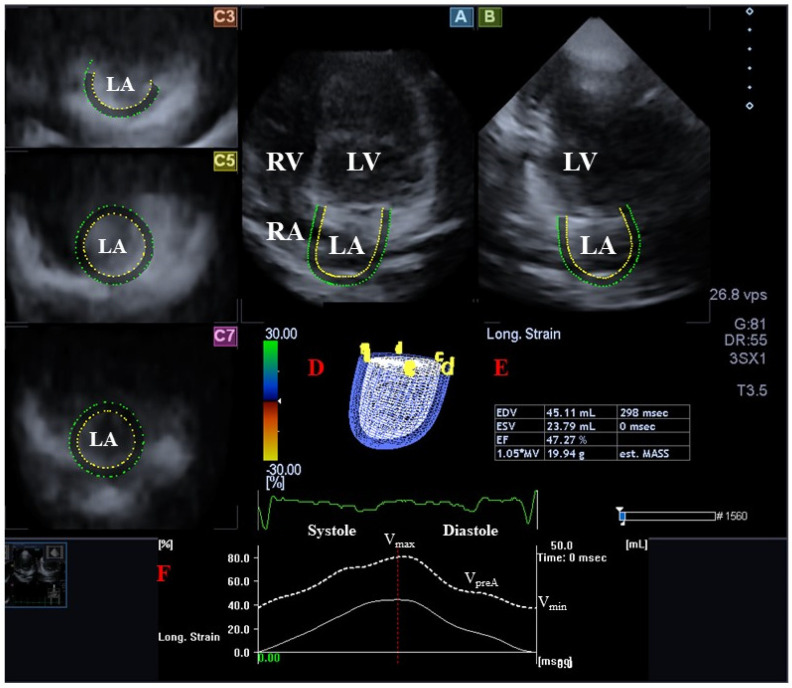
Assessment of the left atrium (LA) via three-dimensional (3D) speckle-tracking echocardiography using apical longitudinal four-chamber (A) and two-chamber (B) views, and short-axis views at basal (C3), midatrial (C5), and superior LA level (C7). Three-dimensional model of the LA (D), calculated LA volumetric data (E), and time— LA global volume changes (white dotted line) during the cardiac cycle are also presented (F) together with maximum (V_max_), preatrial contraction (V_preA_), and minimum (V_min_) LA volumes. Abbreviations: LA, left atrium; LV, left ventricle; RA, right atrium; RV, right ventricle; EDV, end-diastolic volume; ESV, end-systolic volume; EF, ejection fraction; V_max_, end-systolic maximum LA volume; V_preA_, early diastolic pre-atrial contraction LA volume; V_min_, end-diastolic minimum LA volume.
